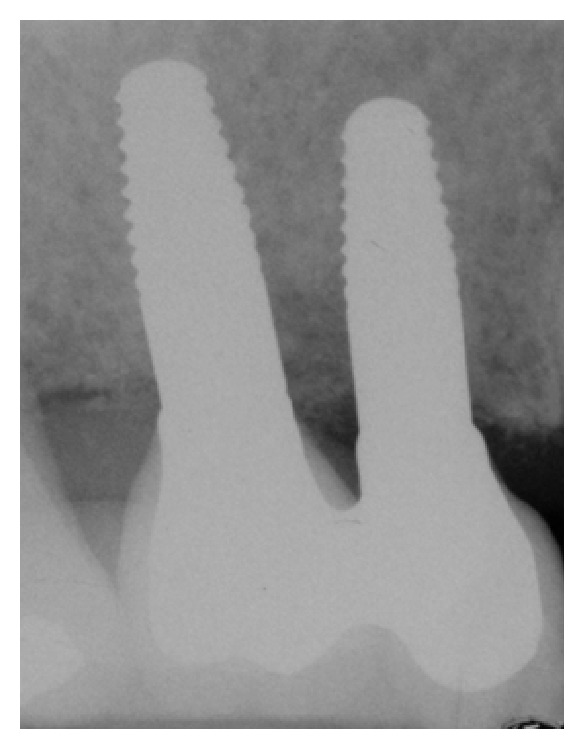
X-ray picture 24 months after surgery.
